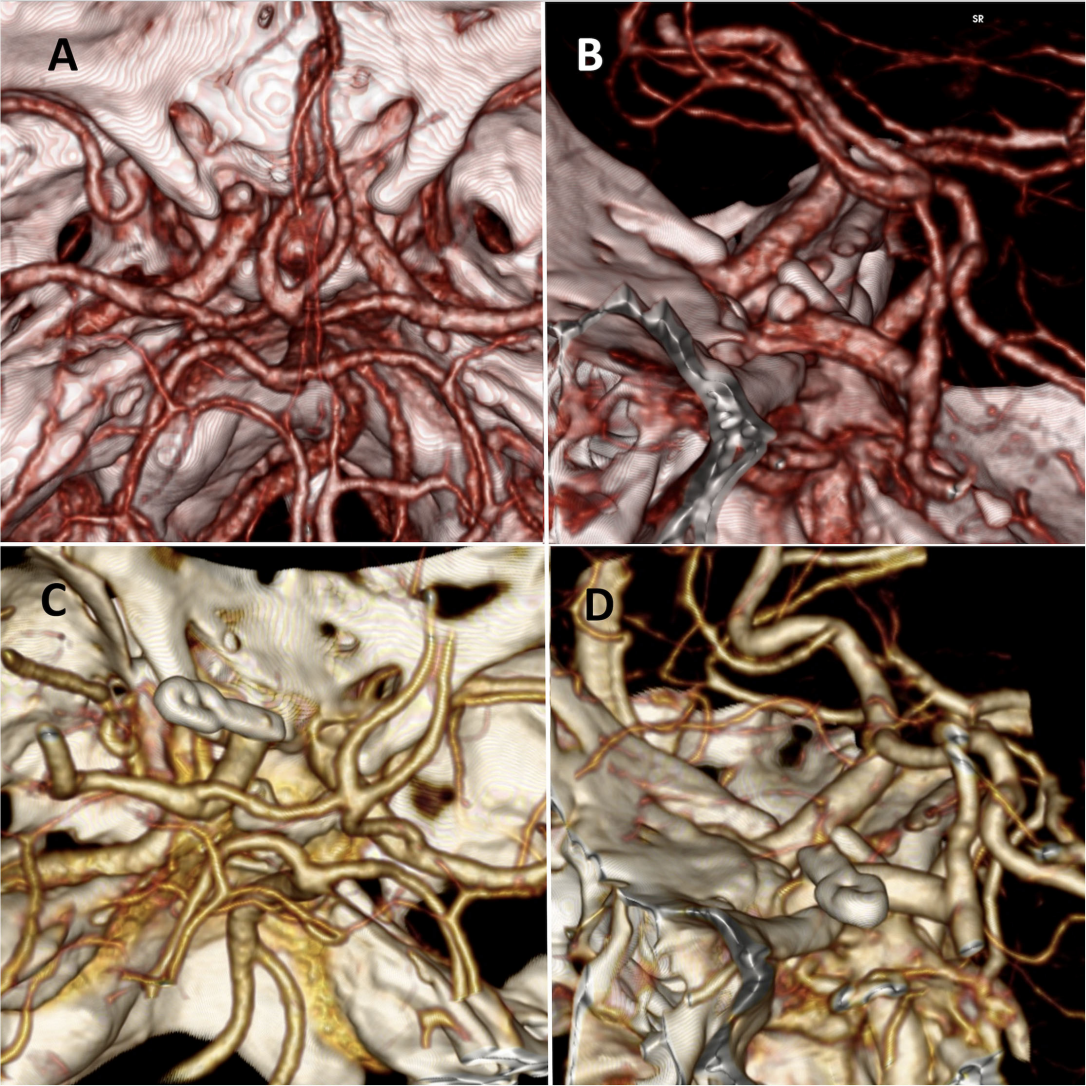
(A,B) Preoperative CT angiography reconstruction of case 1 where we can observe a left superior carotid-ophthalmic aneurysm. (C,D) Postoperative CT angiography reconstruction with and excluded aneurysm after clipping.
